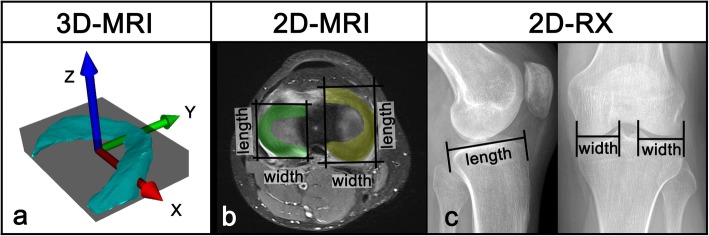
Measurement of meniscal width/length/height. a Width/length/height: meniscal dimensions are measured using an oriented bounding box. The box was aligned to the meniscus roots, and the size was adjusted as long as the entire meniscus body was enclosed. The dimensions of the meniscus correspond to the length of the sides of the box: *y*-axis (green arrow) = meniscal width; *x*-axis (red arrow) = meniscal length; *z*-axis (blue arrow) = meniscal height. b 2D-MRI sizing based on MRI of the contralateral side. c 2D-RX sizing according to Pollard on ipsilateral radiography
